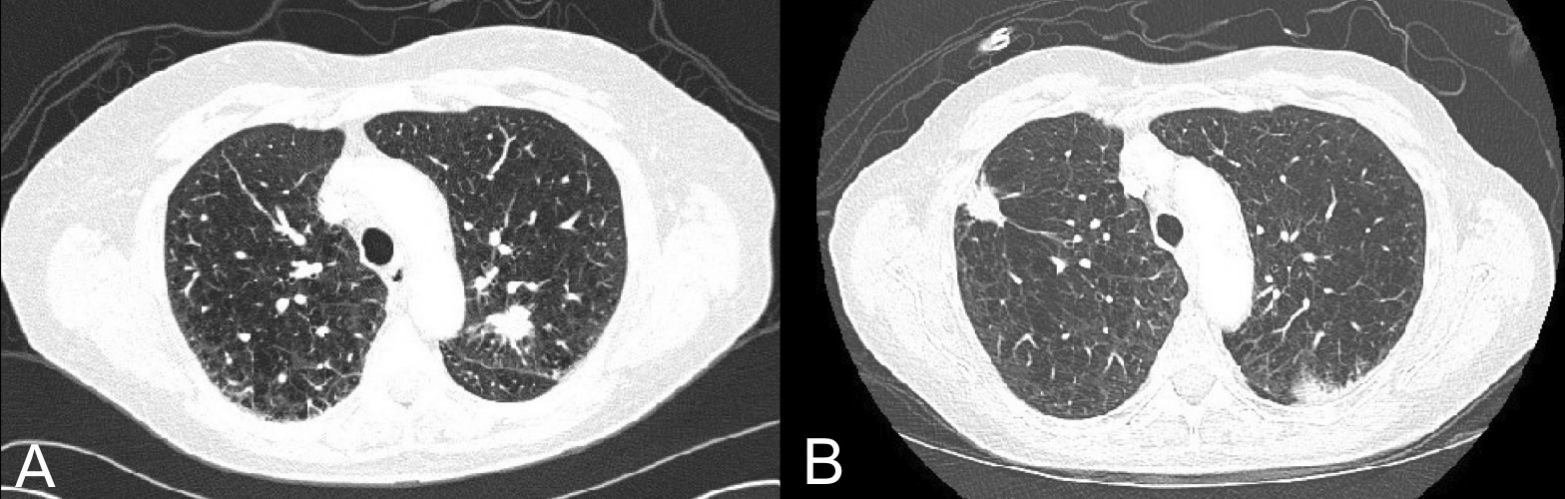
Pre-treatment CT images of (A) LUL LS-SCLC and (B) RUL Stage IA NSCLC.
